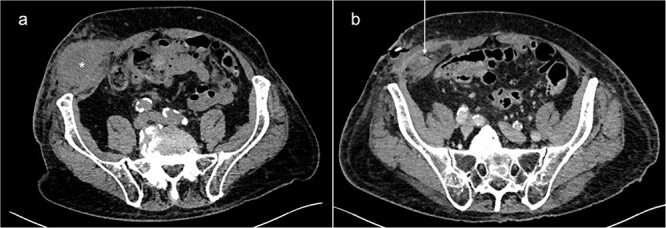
Abdominal CT-scans on initial presentation (a) and day 5 (b). A large fluid collection (*) can be seen in the right lower abdominal wall. After drainage, the appendix (white arrow) could be identified inside the abscess cavity, indicating the existence of a PVH.
